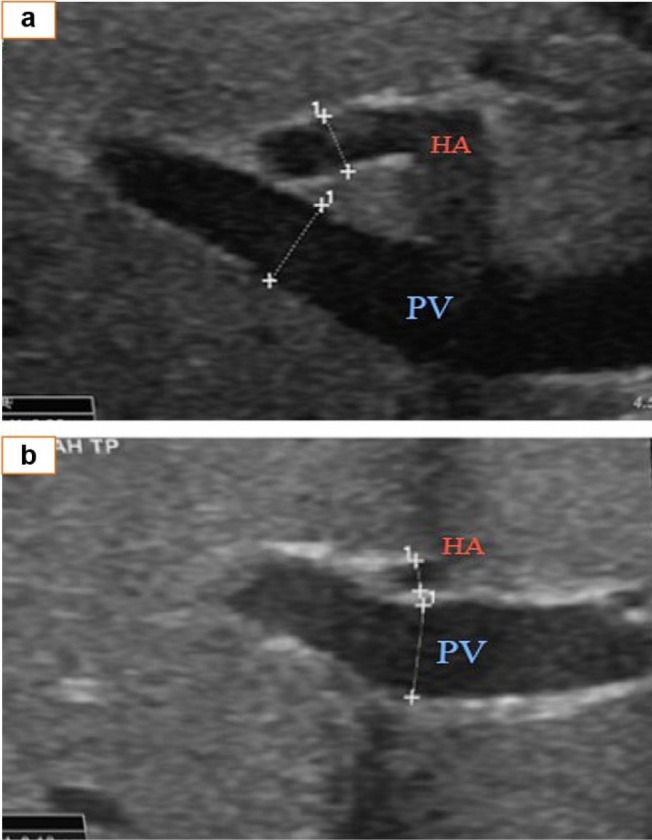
Ultrasound indicating hepatic and portal vein measurements. a, The HA/PV ratio is 0.25/0.38. At approximately 0.66, this ratio is >0.49, suggesting no BA. b, The HA/PV ratio is 0.13/0.39. At approximately 0.33, this ratio is <0.49 and suggests BA. HA: hepatic artery, PV: portal vein, d1: the width of the HA, d2: the width of the PV.
